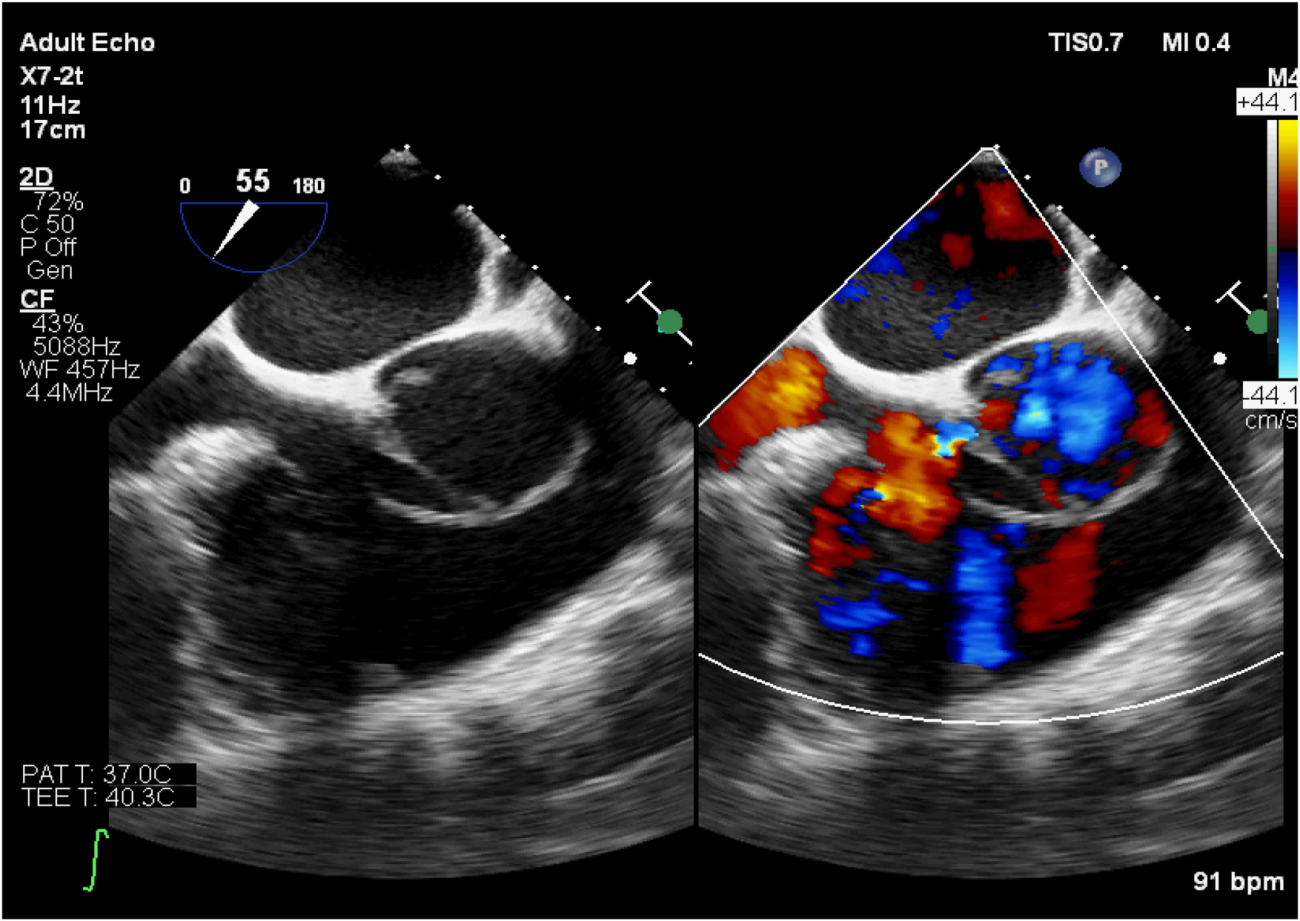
Transesophageal echocardiography (short-axis view of the aortic valve). Left panel: The aortic valve appears tricuspid, with the non-coronary cusp still attached to the annulus; rupture and detachment of the left and right coronary valve annuli are present, with the valve leaflets swinging along with the annuli. Right panel: Severe aortic valve regurgitation is observed.
